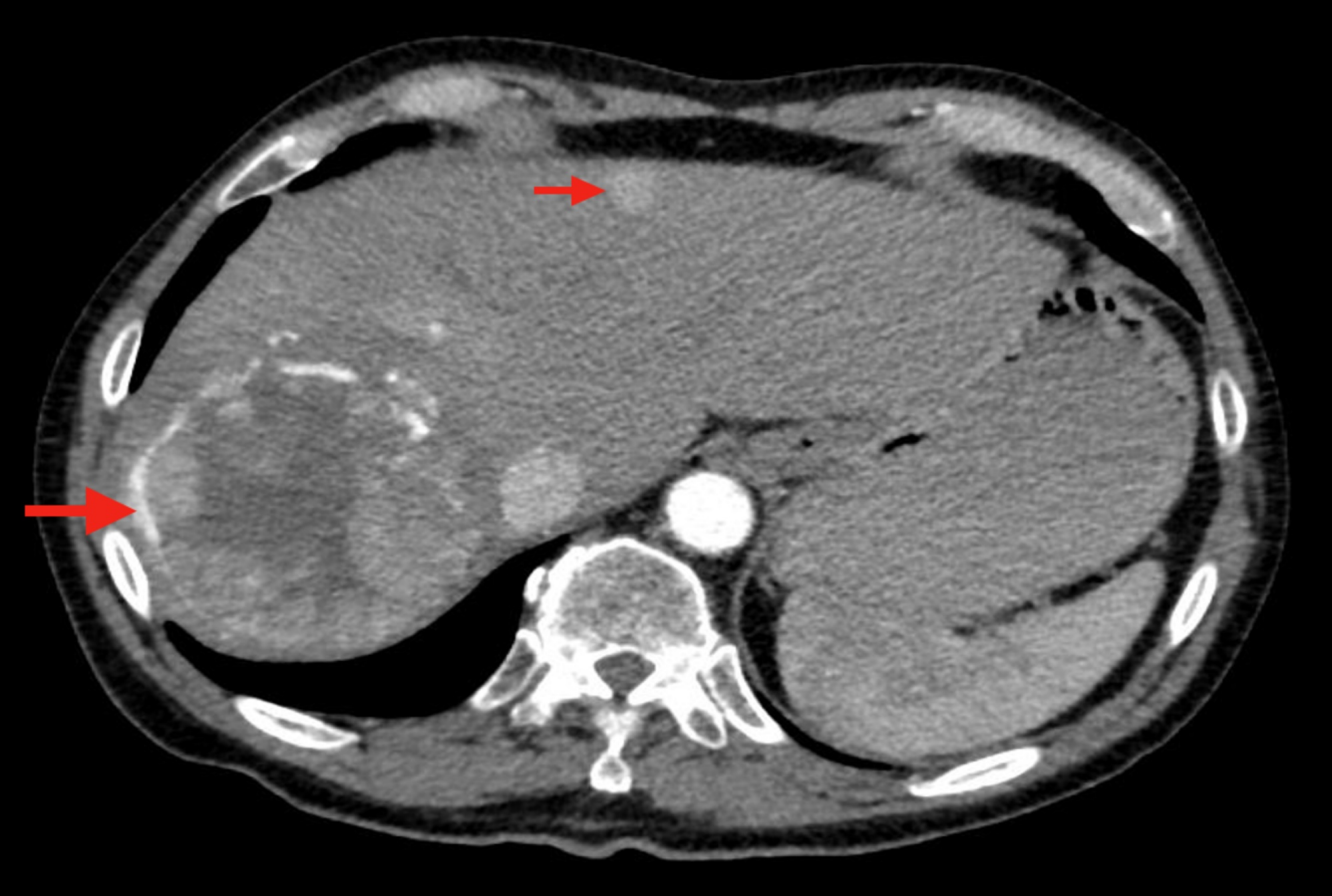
CT imaging 13 months after diagnosis The right hepatic heterogeneous mass (large arrow) demonstrates a central area of necrosis. The hepatic inferior vena cava does not appear to be invaded or compressed. A smaller lesion in the superior lobe of the left liver is also seen (small arrow).
